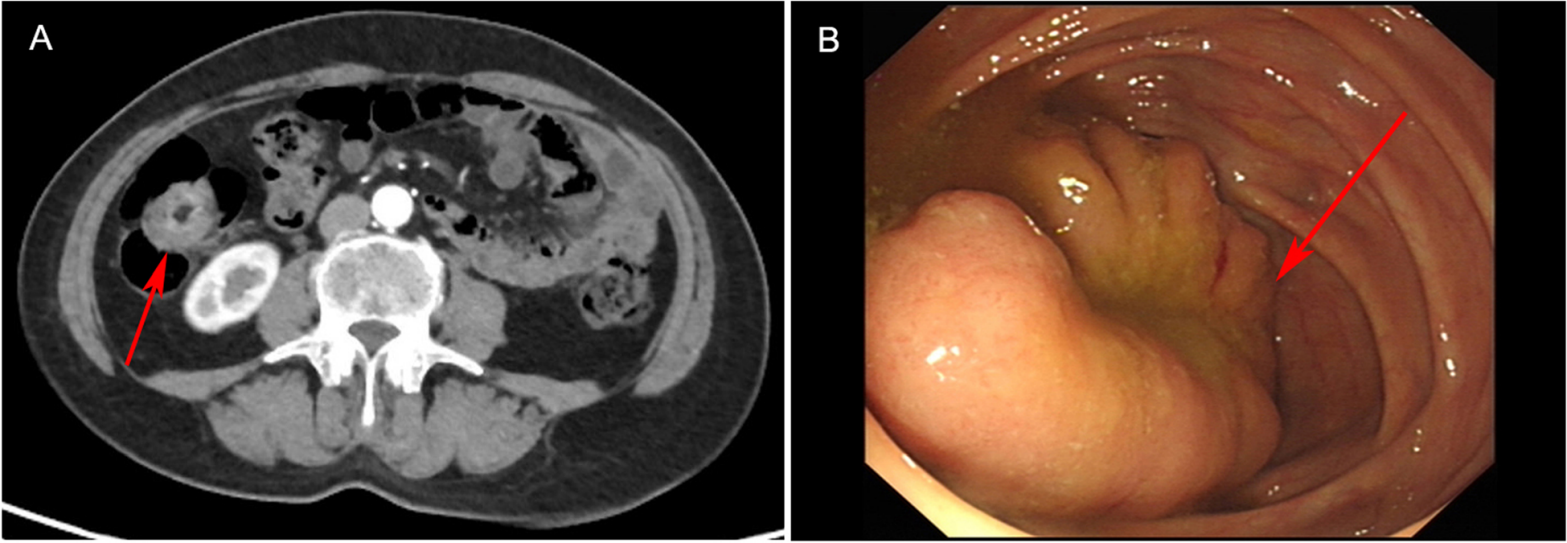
Preoperative findings. a. Computed tomography reveals a 3.5 × 2.5 cm intraluminal protruding mass with central fat component in the cecum. Regional lymph nodes are not enlarged. b. Colonoscopy reveals a long protruding lesion in the cecum, 3.5 cm in size
